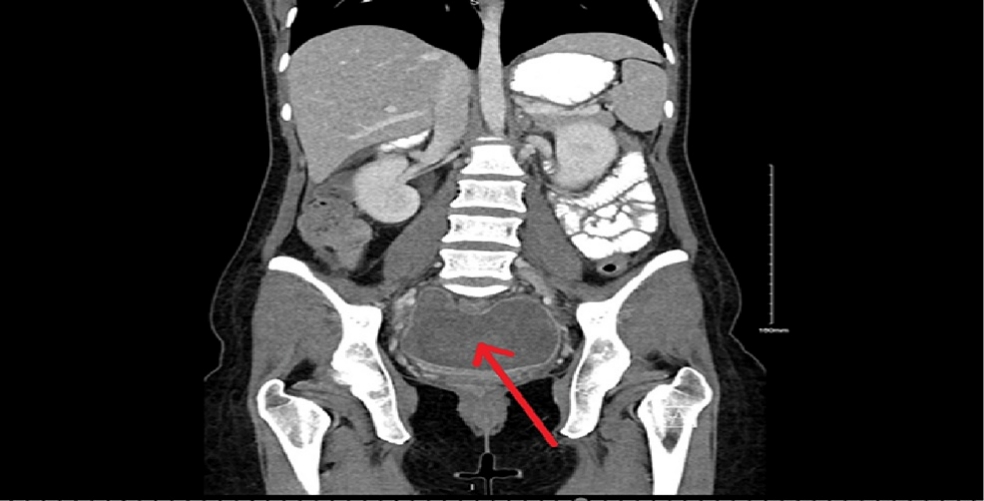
CT coronal view demonstrating a pelvic collection measuring 11.7×6.7×11.1 cm (red arrow) CT: computed tomography
